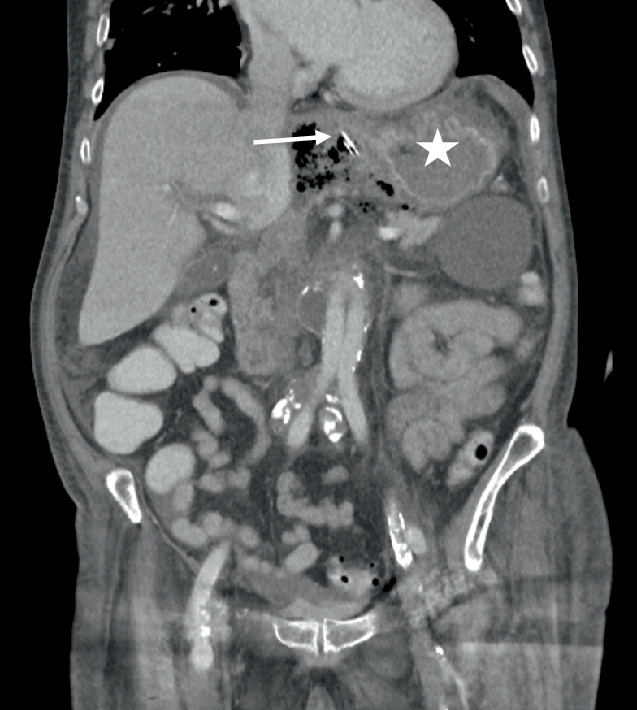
CT-morphologische Darstellung der fehlliegenden NGT (*Pfeil*) mit umgebender Sondenflüssigkeit und Lufteinschlüssen neben dem Magen (*Stern*). (Abbildungsquelle: Aus dem klinischen Image- und Bilddokumentenfokus der Klinik für Radiologie und Nuklearmedizin; Otto-von-Guericke-Universität mit Universitätsklinikum, Magdeburg, Deutschland)
